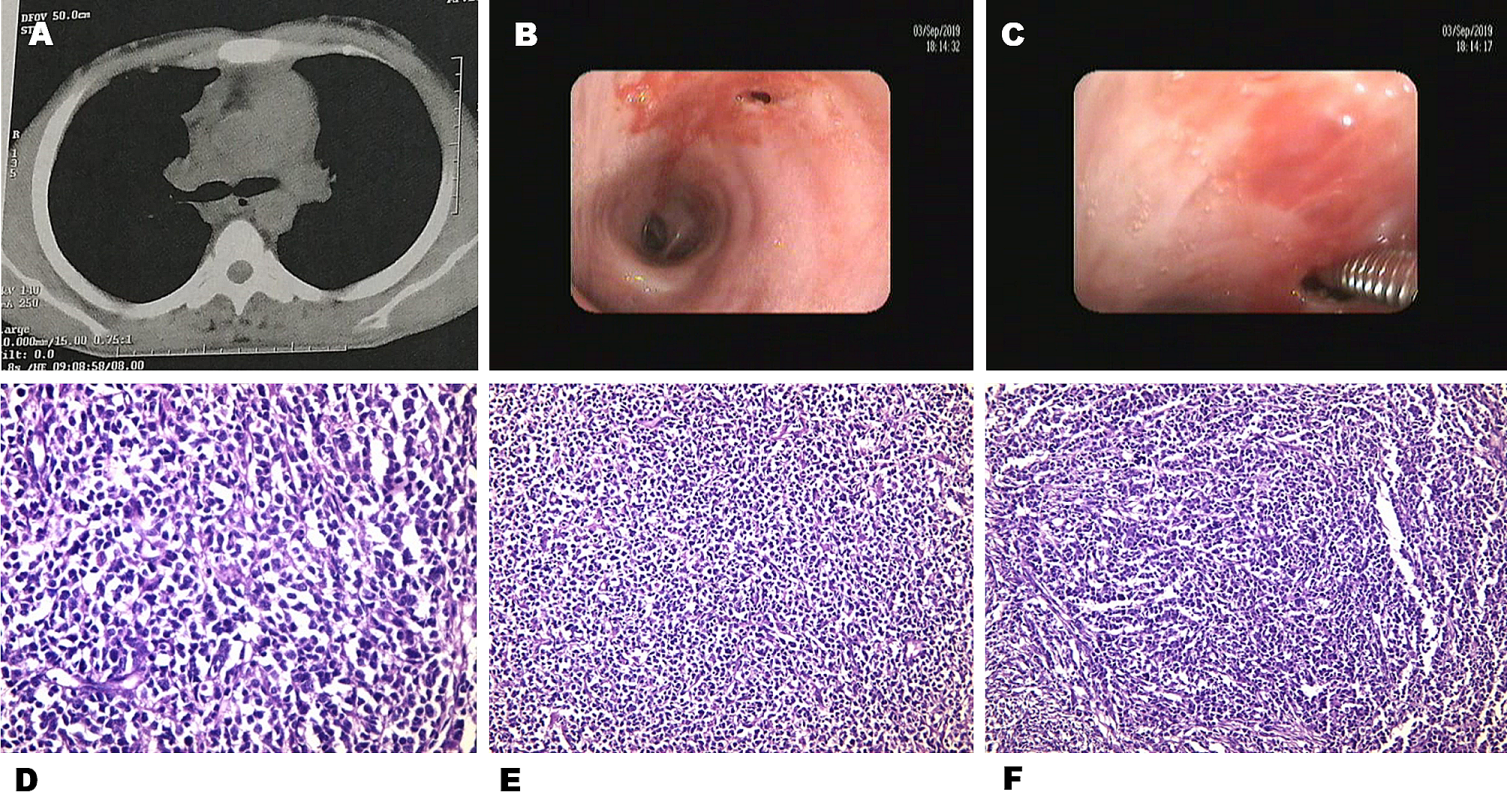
21 years old male, presenting with oedema of the face & upper limb with severe dyspnea. CT chest mediastinal window (A) showing subcarinal & bilateral hilar, discrete mediastinal lymph nodes. The forceps was advanced through the incision made in the bronchial wall (B, C) and LN-TBFBs was obtained from subcarinal L.N. LN TBFB image (high power) (D), (intermediate power) (E) & LN-TBFB image (low power) (F) showing atypical round cells (a case of large cell lymphoma)
